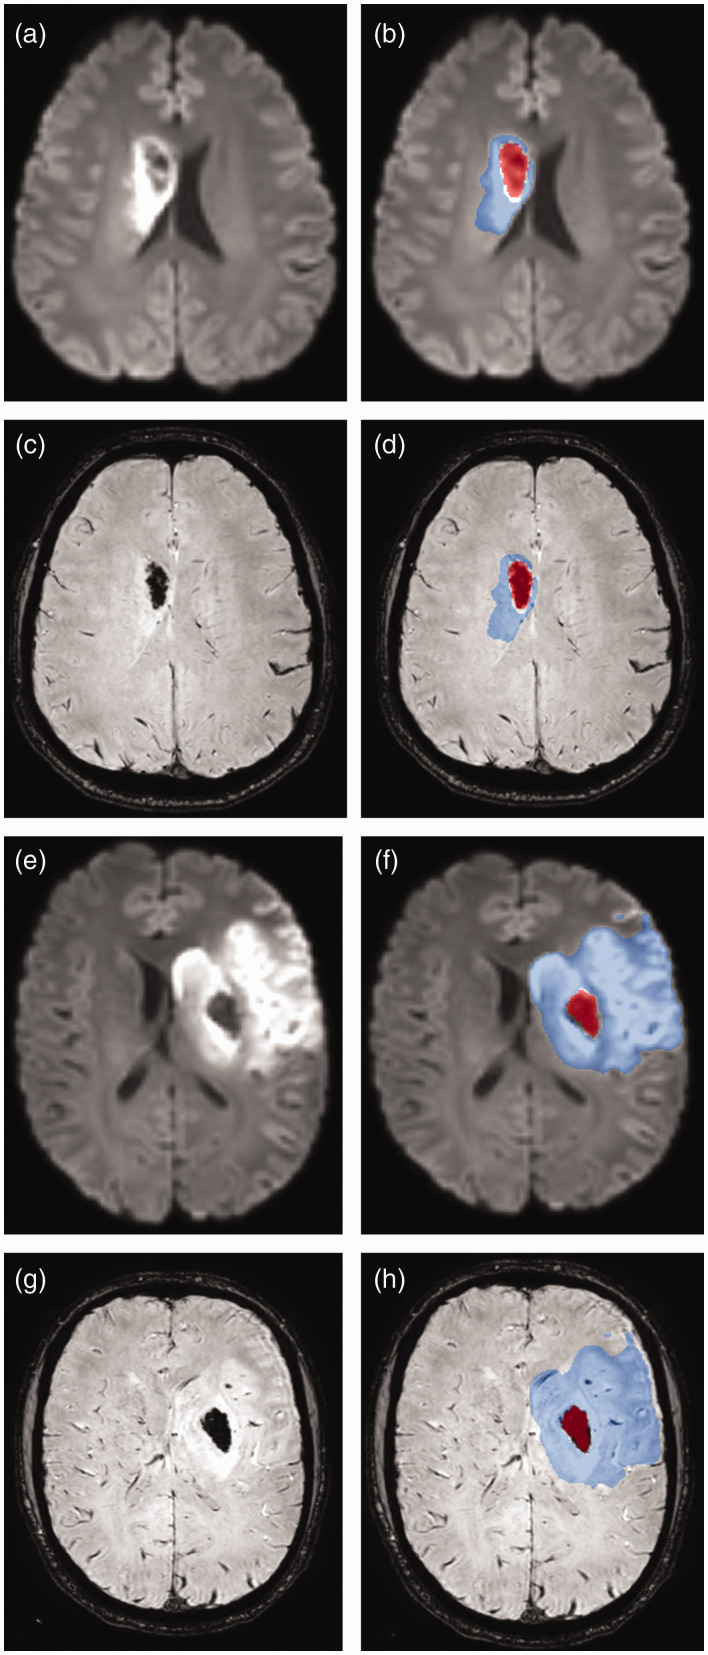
Two exemplary cases of parenchymal hematoma (PH) after thrombectomy. The left column shows co-registered diffusion-weighted imaging (DWI, a, e) and susceptibility-weighted imaging (SWI, c, g) sequences. The right column shows the manually segmented masks for both the infarct (in blue; infarct segmentations of DWI-positive ischemic lesions) and the hematoma (in red) overlaid onto DWI (b, f) and SWI (d, h) sequences. The second case (bottom two rows, e–h) is the same as in Figure 2.
